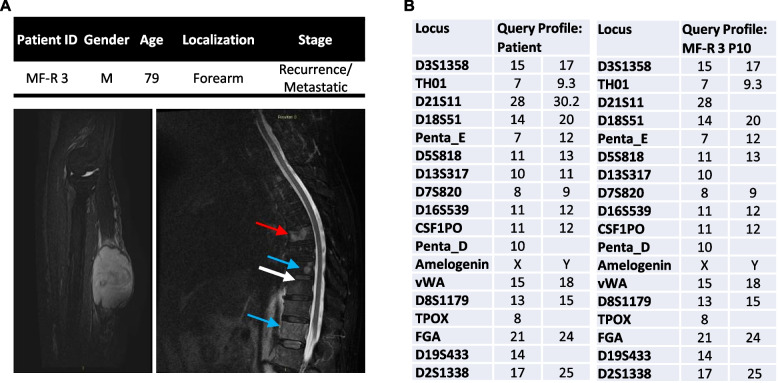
Patient’s data and generation of MFS derived cell line. A Summary of clinical data of the patient (top), Sag T2 FAT Sat MRI of the forearm shows the recurred tumour mass (bottom left), while Sag T2 FAT Sat MRI of the spine (bottom right) shows multiple hyperintense metastatic lesions (blue arrows) with a pathological fracture in T6 (red arrow). B STR profiling data obtained by ATCC of the patient’s tumour sample (left) and MF-R 3 cells at passage 10 (hypothesized immortalization passage) (right)
